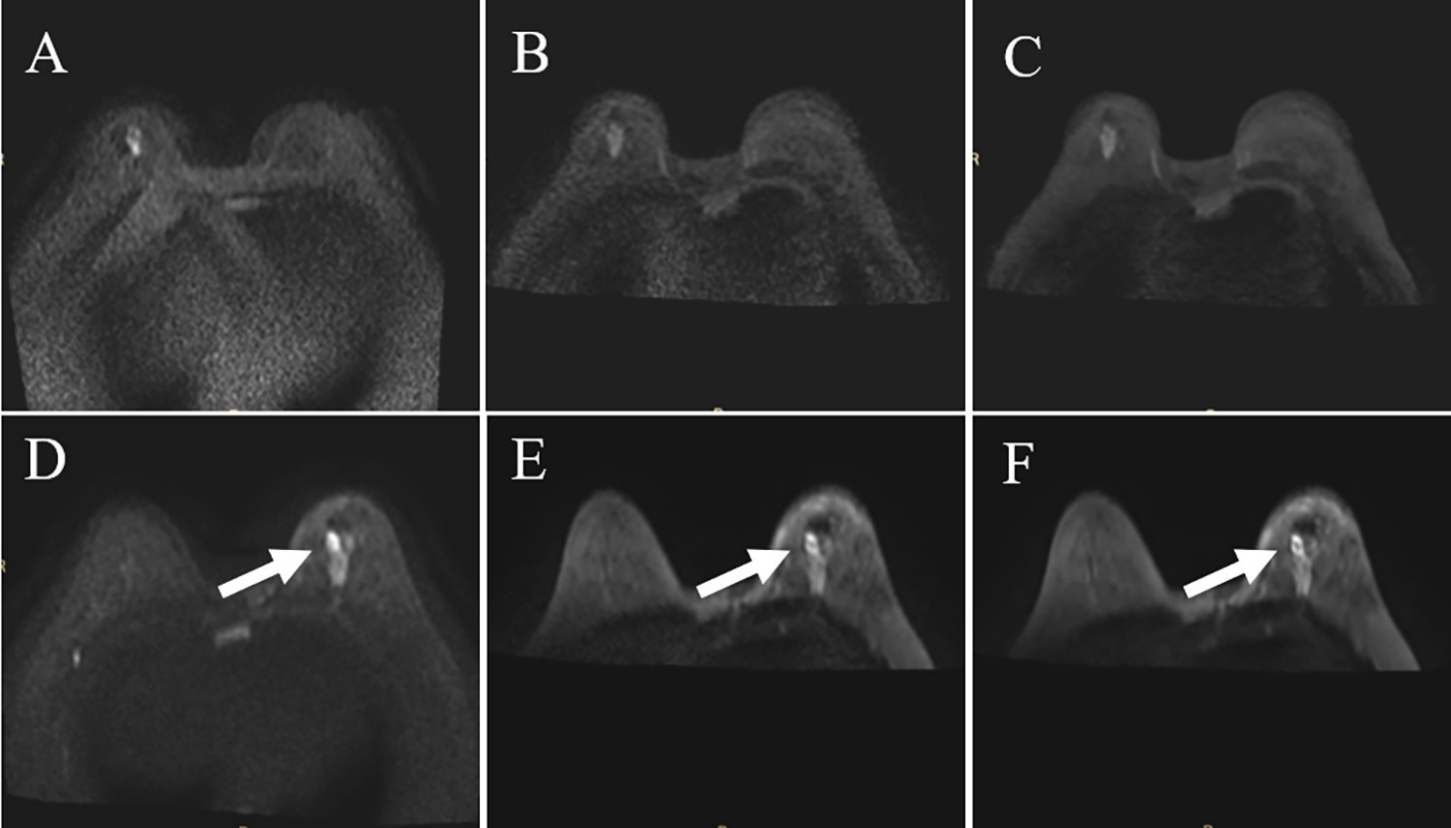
Images used for subjective quality assessment. Conventional (A), FOCUS (B), and FOCUS-DLR (C) DWI of a 64-year-old patient with suspected invasive breast cancer in the right breast. The overall image quality of FOCUS DWI was superior to that of conventional DWI and inferior to that of FOCUS-DLR DWI. Conventional (D), FOCUS (E), and FOCUS-DLR (F) DWI of a 49-year-old patient with suspected invasive cancer in the left breast. FOCUS (E) and FOCUS-DLR (F) DWI are superior to conventional DWI (D) in lesion conspicuity and anatomical detail, where the white arrow indicates the lesion. DLR, deep-learning-based reconstruction; DWI, diffusion-weighted imaging; FOCUS, field-of-view optimized and constrained undistorted single-shot.
